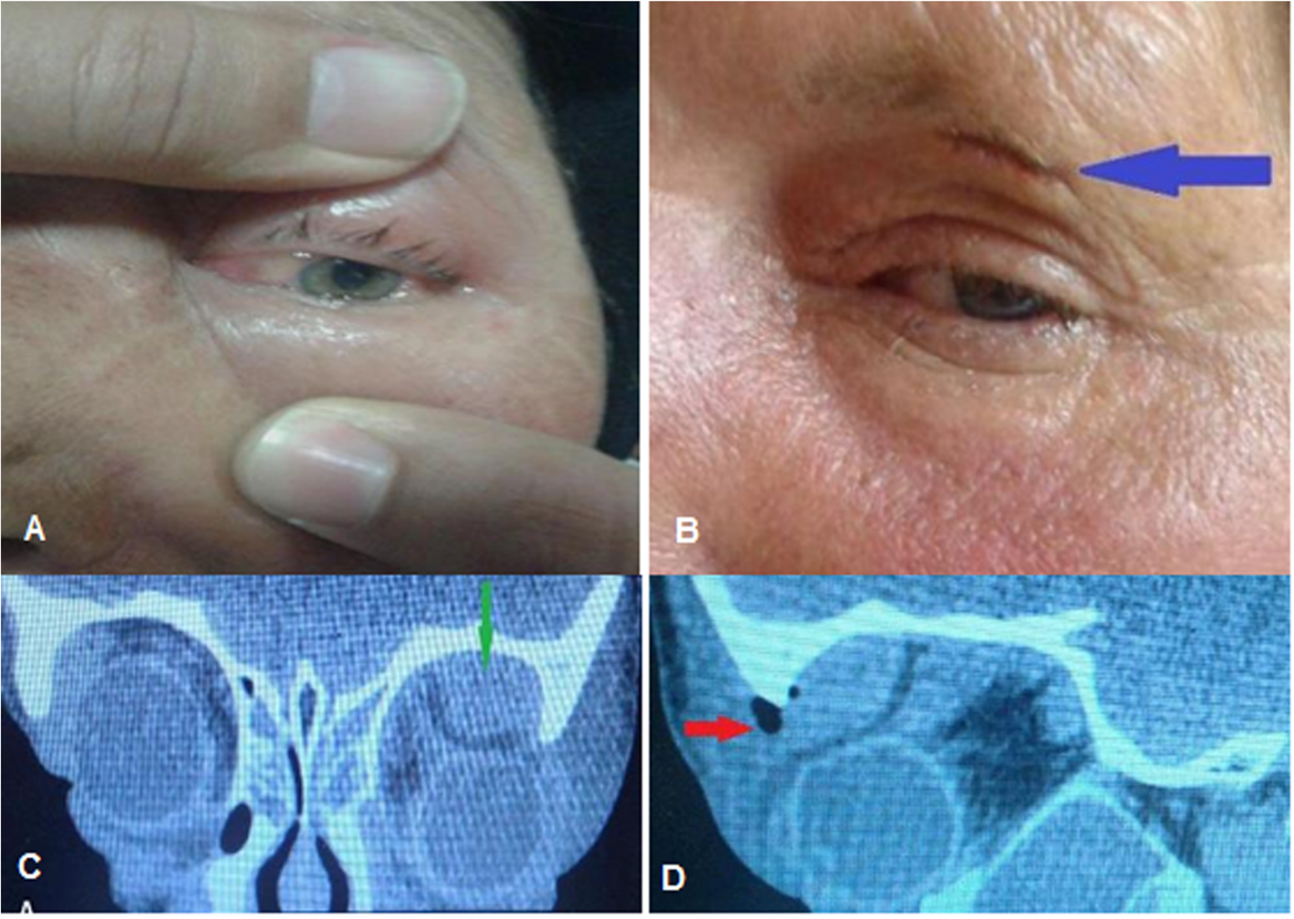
A 62-year-old woman with visual impairment in presenting time, a external eye exam shows upper lid swelling and redness with limitation of motion and conjuctival chemosis, b 5 day after external abscess drainage with sub-brow incision (blue arrow), c orbital CT scan coronal view revealed a left supra-temporal subperiostal abscess (green arrow), d and sagittal view showed a superior abscess with hypodense area (red arrow) and compression effect to the globe
